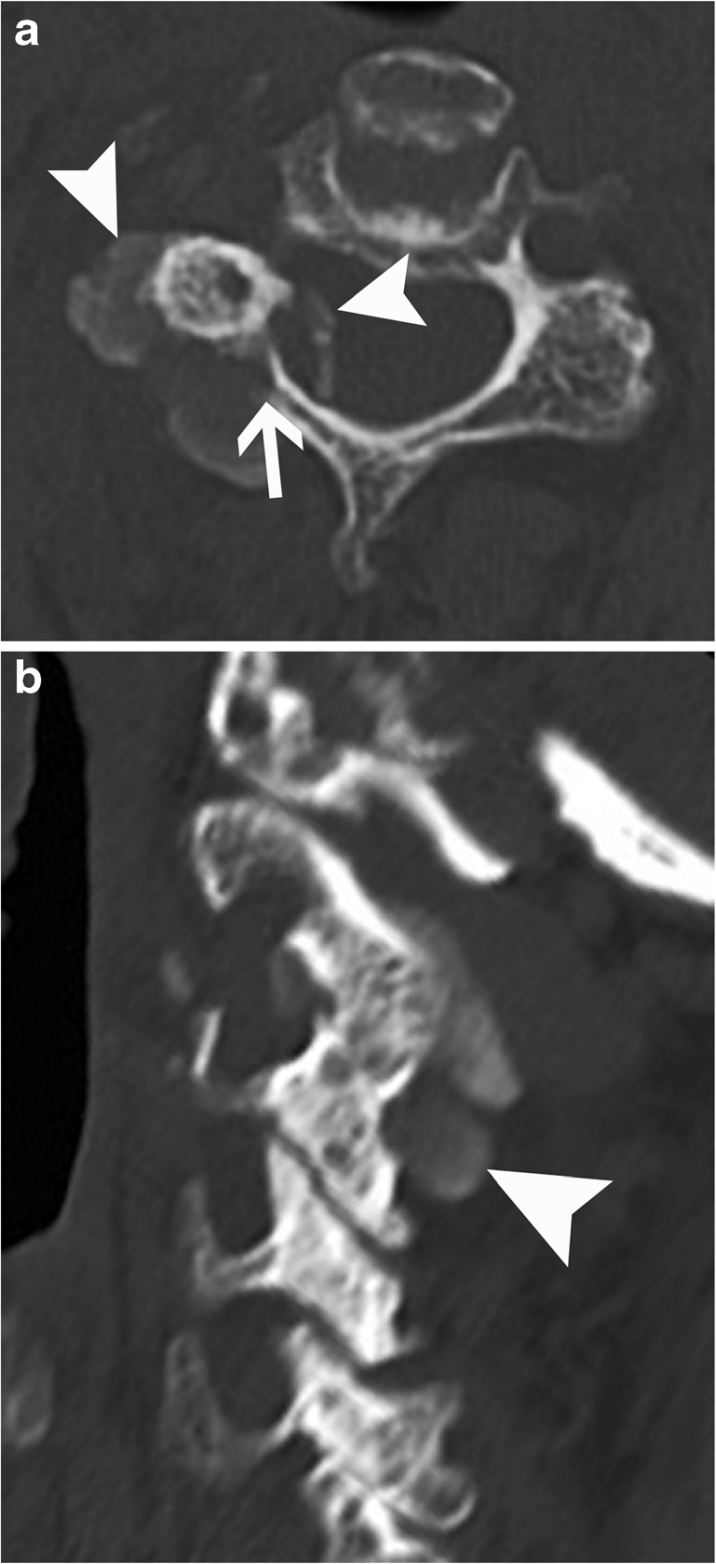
Cervical facet joint calcifications. (a) Axial and (b) sagittal CT images of the cervical spine show dense material (arrowheads) in the right C2–C3 facet joint. Note the associated bone erosion of the right lamina (arrow)
